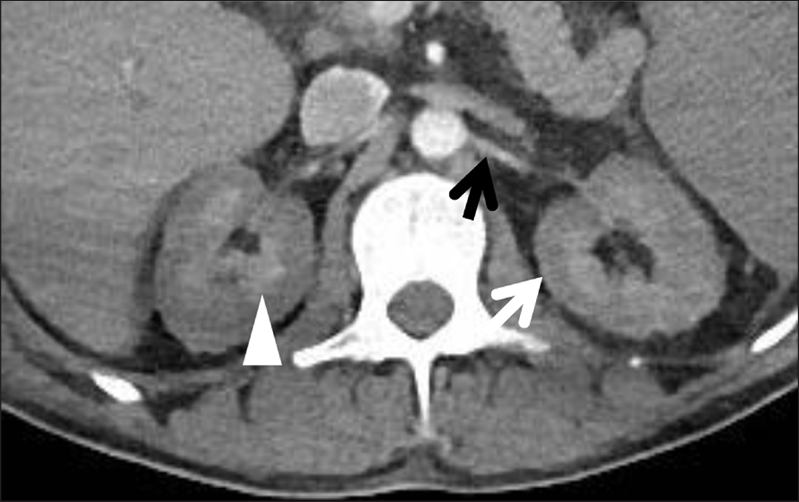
Axial contrast enhanced CT in the arterial phase shows a non-enhancing renal cortex with a capsular enhancing rim (white arrow) and enhancement of the renal medulla (arrowhead). Also note the fine caliber of the renal artery (black arrow).
